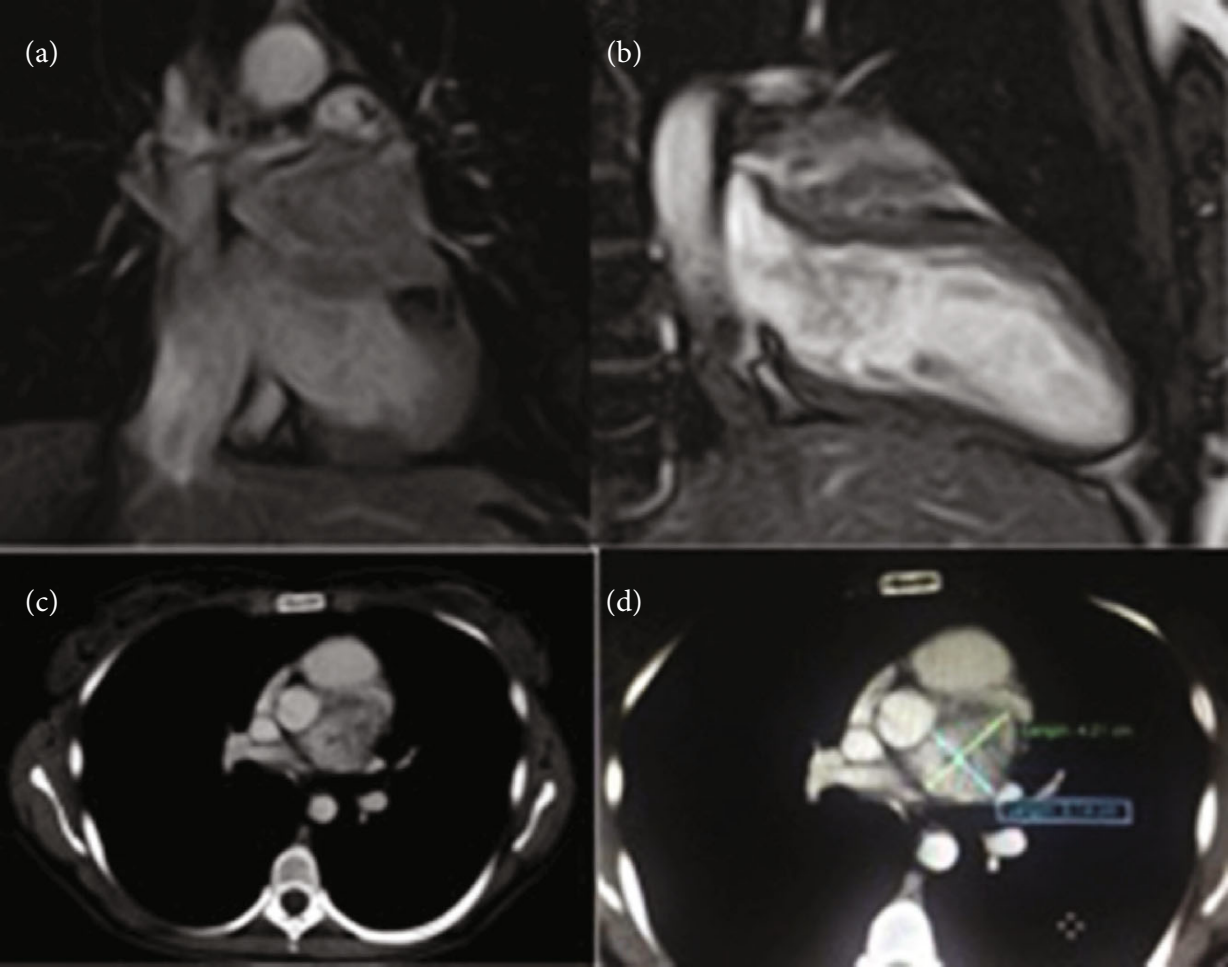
An example of Cardiac MRI with a cardiac paraganglioma [10]. (a) Coronal image with mass (isointense) in the area of the left atrium. (b) Two camber longitudinal view with the mass (isointense) in the left atrioventricular groove. (c, d) Axial view demonstrating the mass in relation to the aortic root (c) and pulmonary artery (d), respectively.
